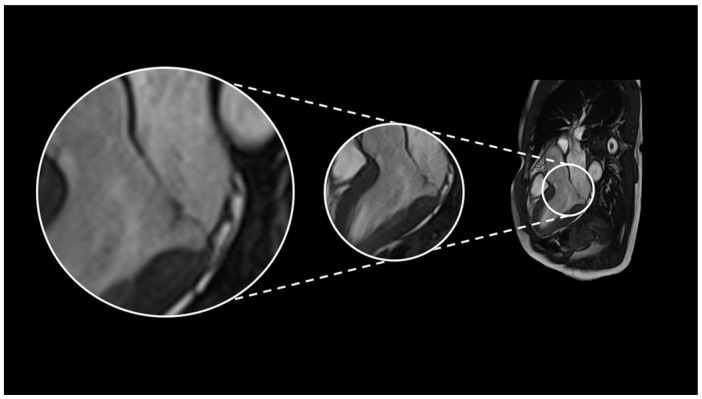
Cardiac magnetic resonance imaging (long-axis three-chamber view) demonstrates mildly redundant, prolapsing mitral-valve leaflets with a mitral annular disjunction (MAD) measuring 4 mm. Cine CMR sequences further reveal curling of the basal inferolateral and anterolateral left-ventricular walls, accompanied by mild mitral regurgitation.
